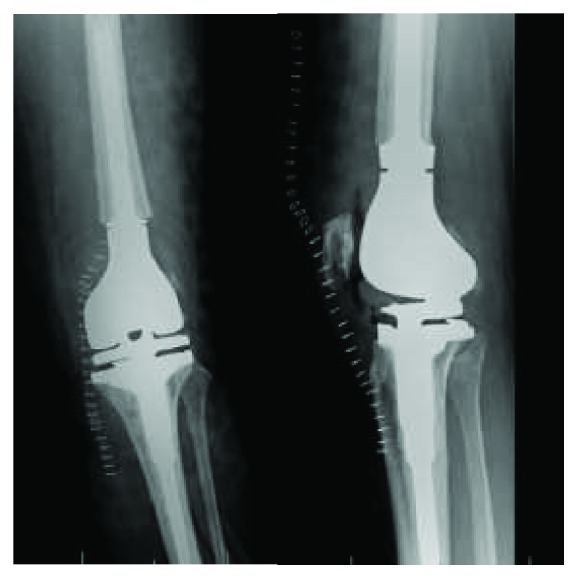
Immediate postoperative radiographs following hinged distal femoral replacement.
